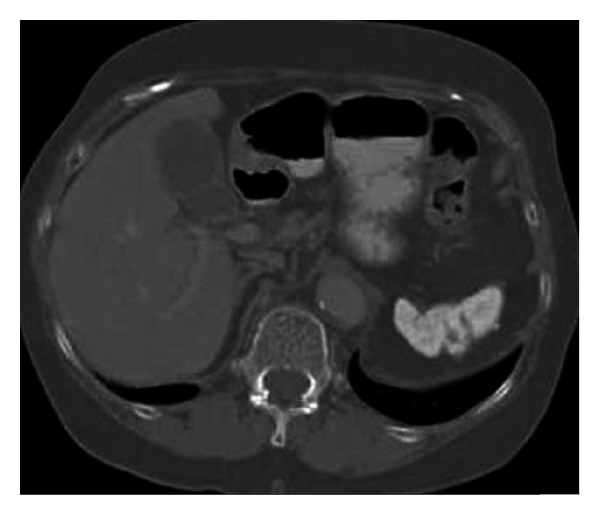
Axial contrast enhanced CT image at bone window demonstrates small and calcified spleen of a patient with sickle cell disease.
